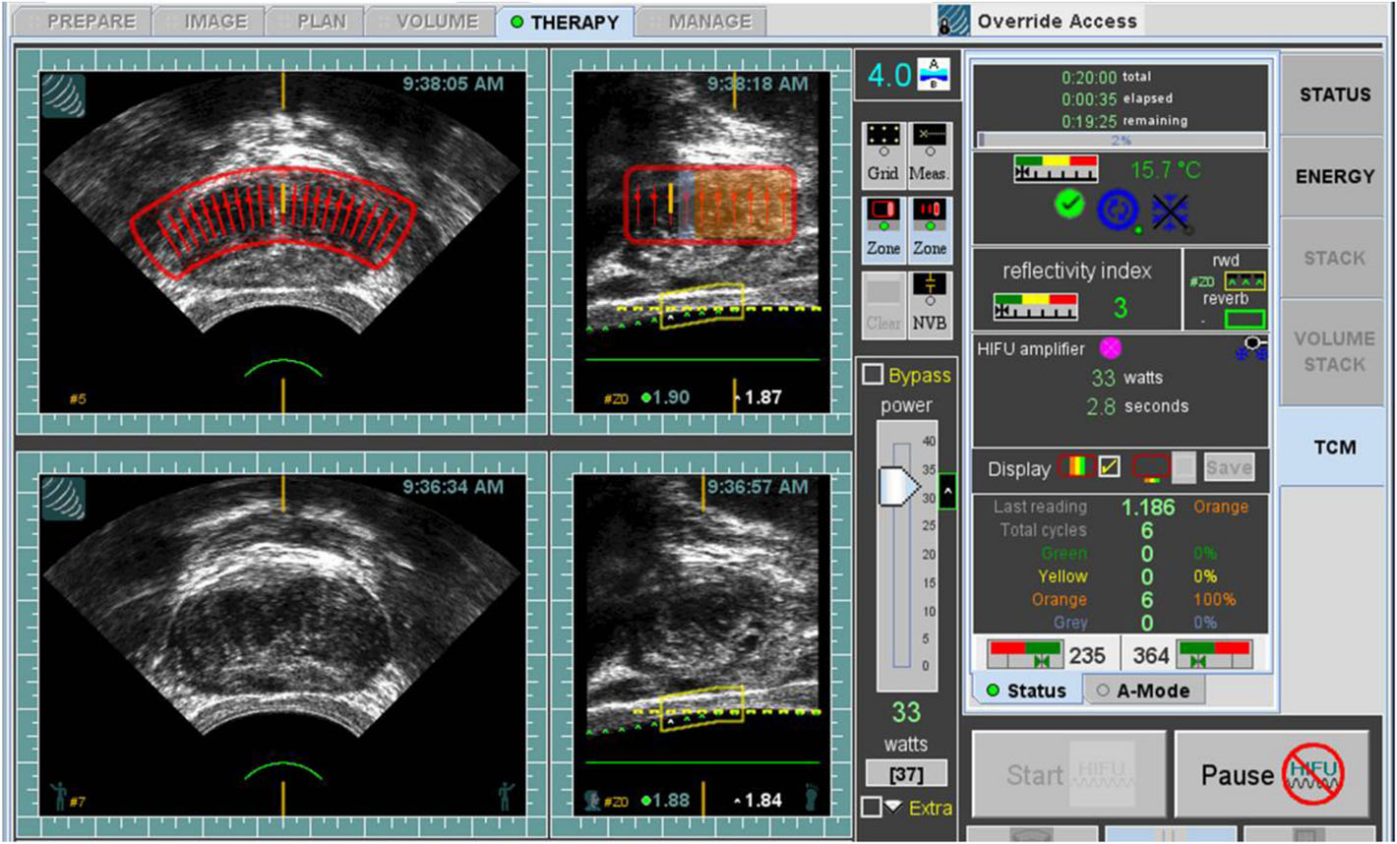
The Sonablate 500 TCM monitor display during the whole gland ablation of prostate tissue. The lower (reference) and upper (real-time) panels are transverse and sagittal ultrasound images of the prostate (pre and post HIFU) for each ablative site, respectively. The upper panel images are marked with transducer focal zone and selected tissue ablative sites. The ΔE calculation is computed after each HIFU exposure and a green, yellow or orange color is overlaid on the ablated site as per Table 1 color scheme
